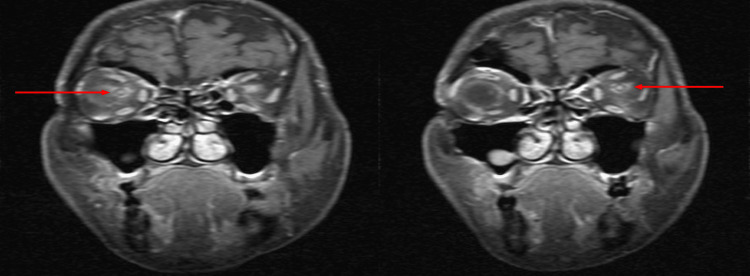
MRI orbits: the orbit T1-weighted fat-suppressed coronal view. Note that red arrows show the classical “doughnut sign” representing optic nerve sheath enhancement.
